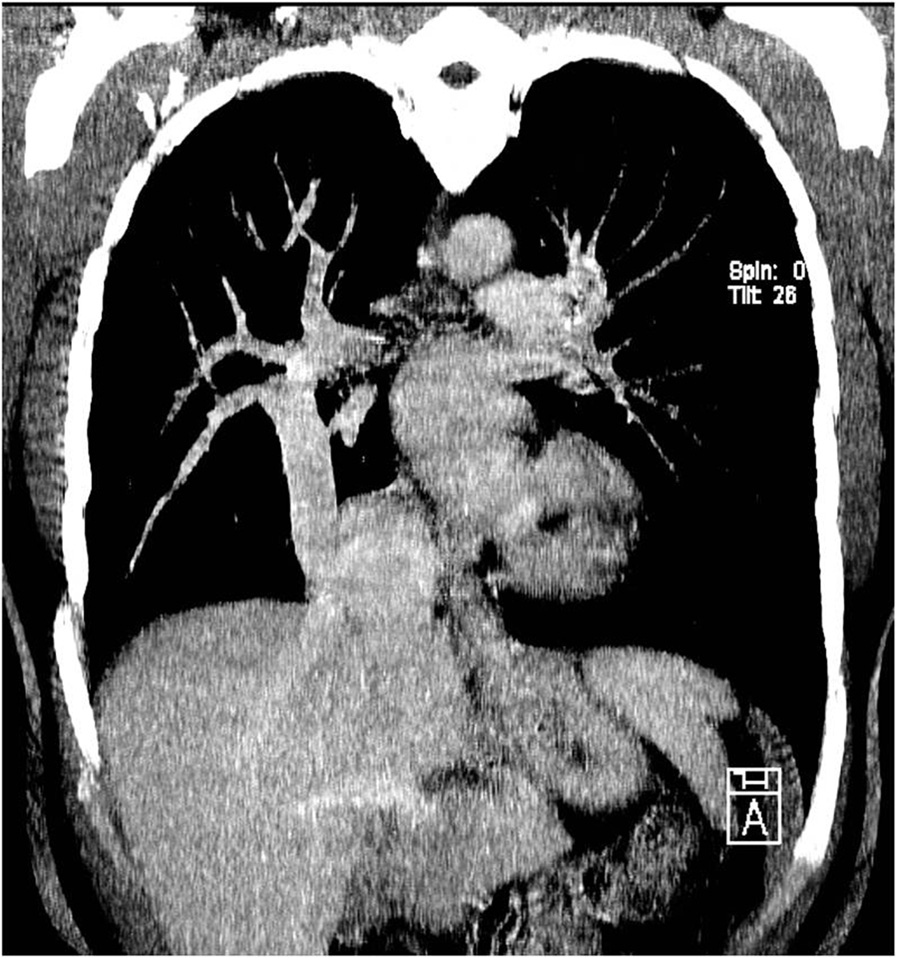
Scimitar sign. Coronal contrast-enhanced computed tomography shows the anomalous drainage of the right pulmonary vein into the inferior vena cava, representing the scimitar sign on conventional radiography.
